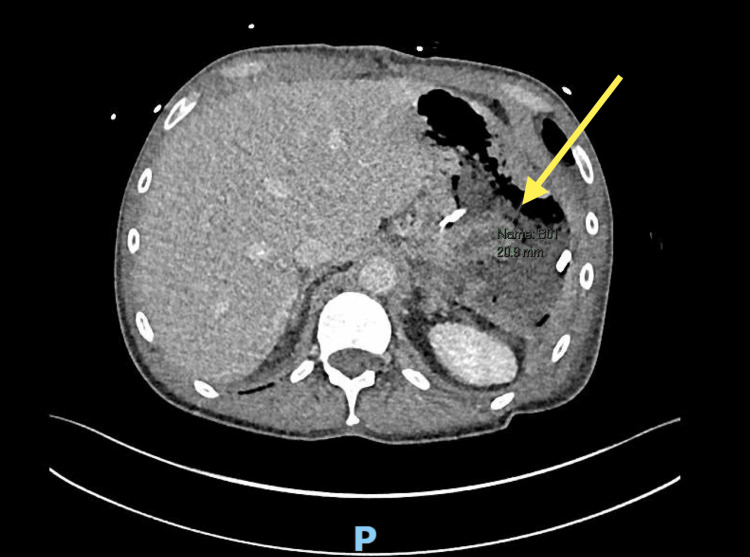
CT abdomen demonstrating a large 21 mm defect in the greater curvature of the stomach (yellow arrow).
